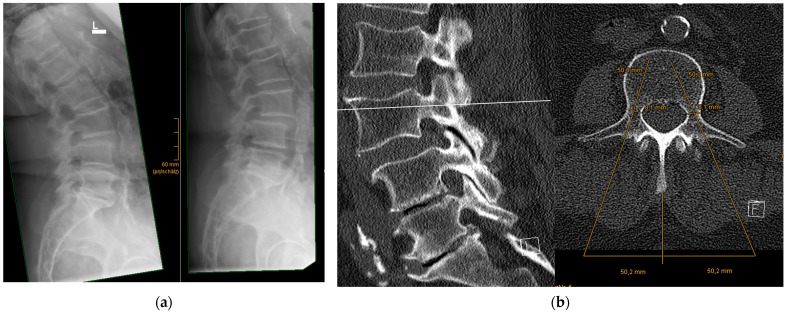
(a) Exemplary case of a 79-year-old woman with unstable spondylolisthesis at L3–L4 causing intractable pain unresponsive to conservative treatment. Consequently, MIS TLIF of L3–L4 was indicated. (b) The preoperative CT-scan in sagittal (left) and axial (right) cross sections, displaying the measured trajectory, convergence angles, and screw dimensions (here, 6.5 mm × 50 mm), as well as the lateral incision point of 5 cm.
